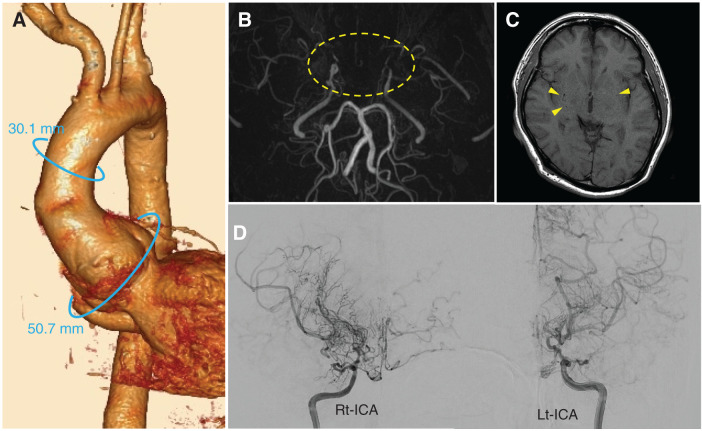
Preoperative findings. (A) Preoperative cardiac CT showed a sinus of Valsalva aneurysm, with no enlargement of the ascending aorta. (B) MR angiography revealed occlusion of bilateral ICAs, with no visualization of the circle of Willis, ACA, or MCA (dotted area). (C) T1-weighted images revealed small flow voids in the basal ganglia, indicating abnormal vascular networks (arrowhead). (D) Selective internal carotid angiography showed poor visualization of the circle of Willis, including ACA and MCA, and the presence of moyamoya vessels. ACA, anterior cerebral artery; ICA, internal carotid artery; Lt, left; MCA, middle cerebral artery; MR, magnetic resonance; Rt, right
